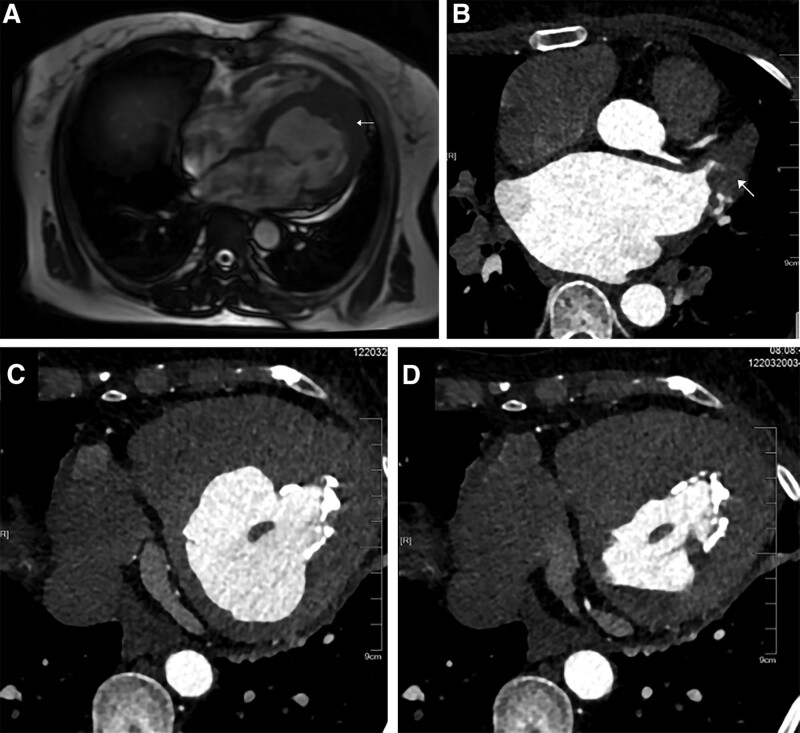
Cardiac CT showing apical calcification (A, white arrow), contrast-enhanced imaging showing apical hypertrophy and filling defect (B, white arrow), and limited left ventricular filling during diastole and systole (C, D). CT = computed tomography.
